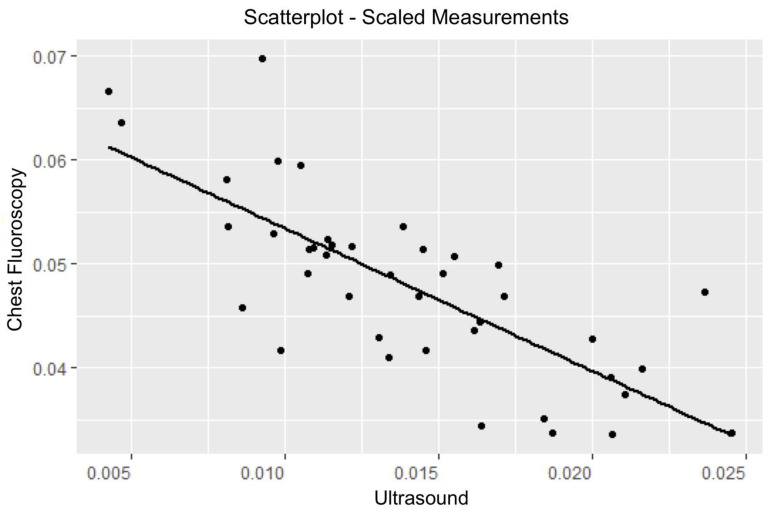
Scatter plot of scaled chest fluoroscopy vs. scaled ultrasound measurements.
